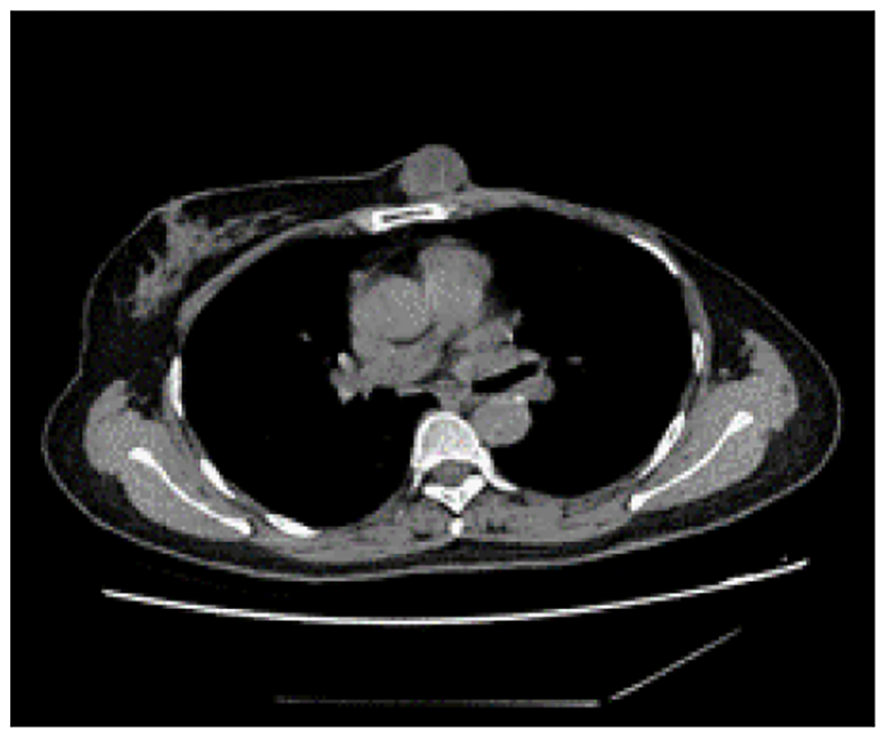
Computed tomography of the thorax identified a subcutaneous mass anterior to the sternum, measuring approximately 3.0 cm x 2.5 cm, characterized by smooth margins and regular morphology.
